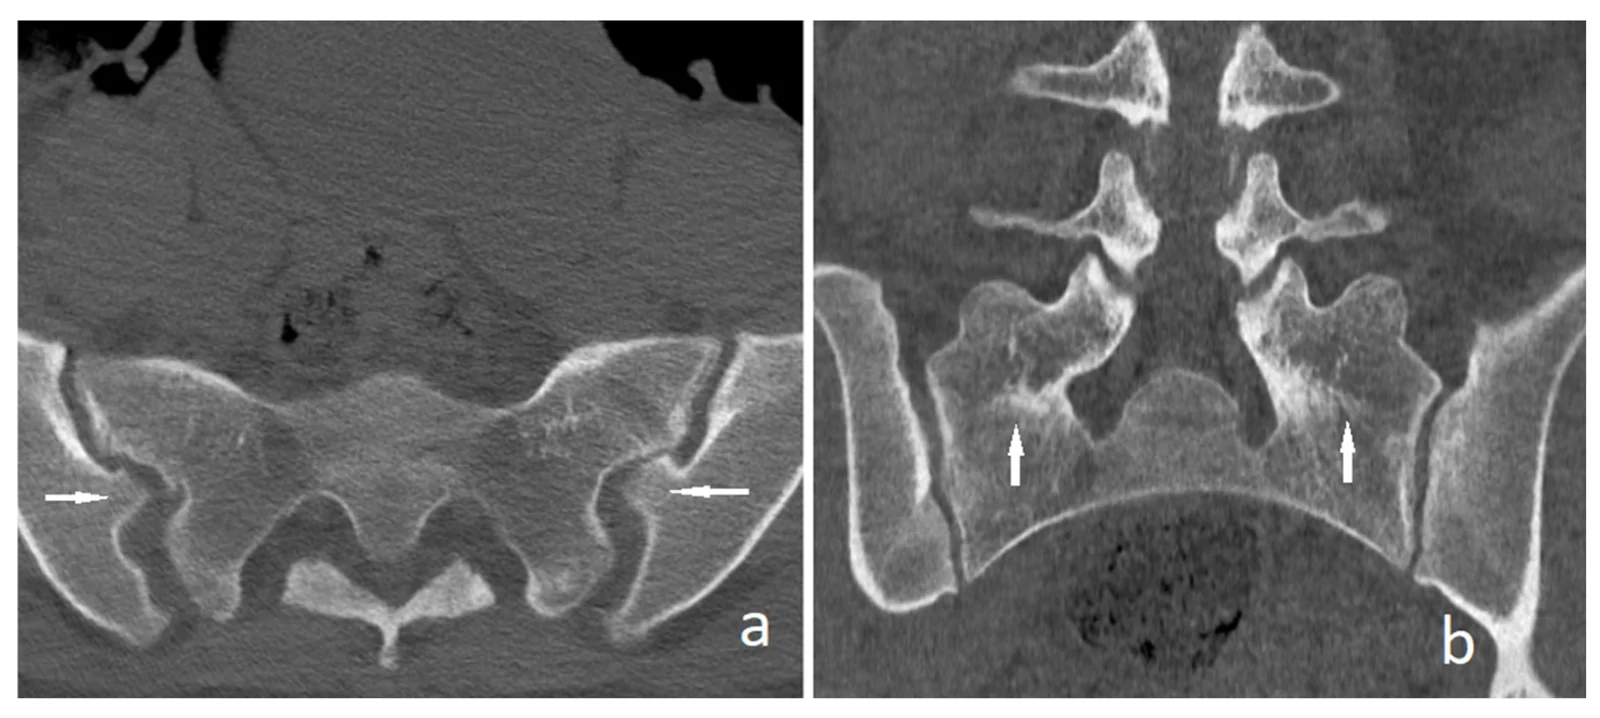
Coronal computed tomography sections through the sacroiliac joints (a) and vertebral column (b) of a 19-year-old female who presented with trauma and had no fracture detected on computed tomography imaging show a bilateral iliosacral complex variant and Castellvi type 3b transitional vertebra, respectively (arrows).
